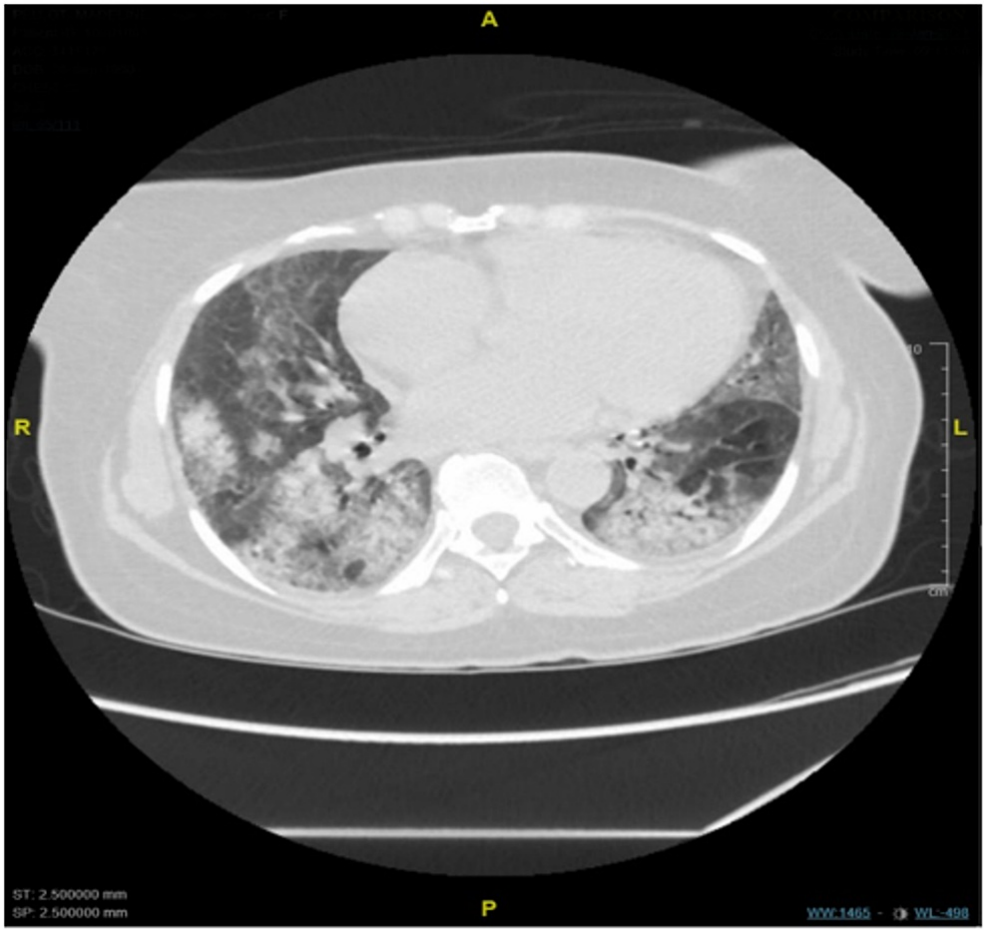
CT chest without contrast showing an abnormal appearance of the parenchyma bilaterally compatible with a crazy paving pattern
